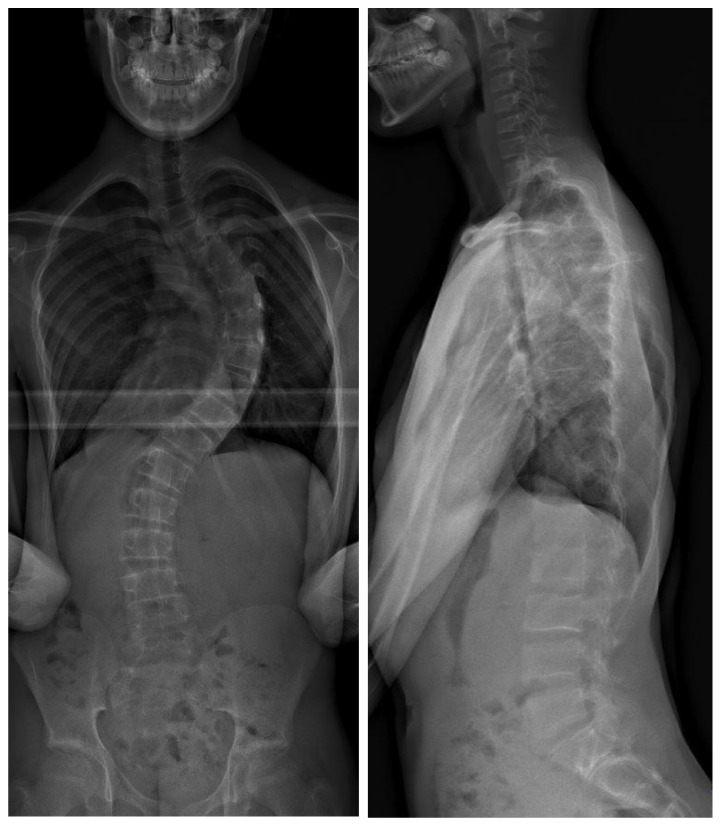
Preoperative biplanar radiograph of a 15-year-old patient with 62° thoracic scoliosis (Lenke I).
